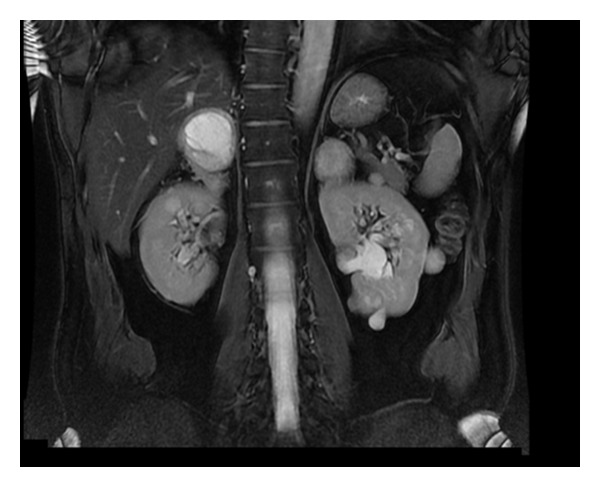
T2-weighted MR image in the coronal plane demonstrating bilateral, adrenal masses and renal cysts. The large adrenal mass on the right side has almost completely replaced the adrenal tissue.
